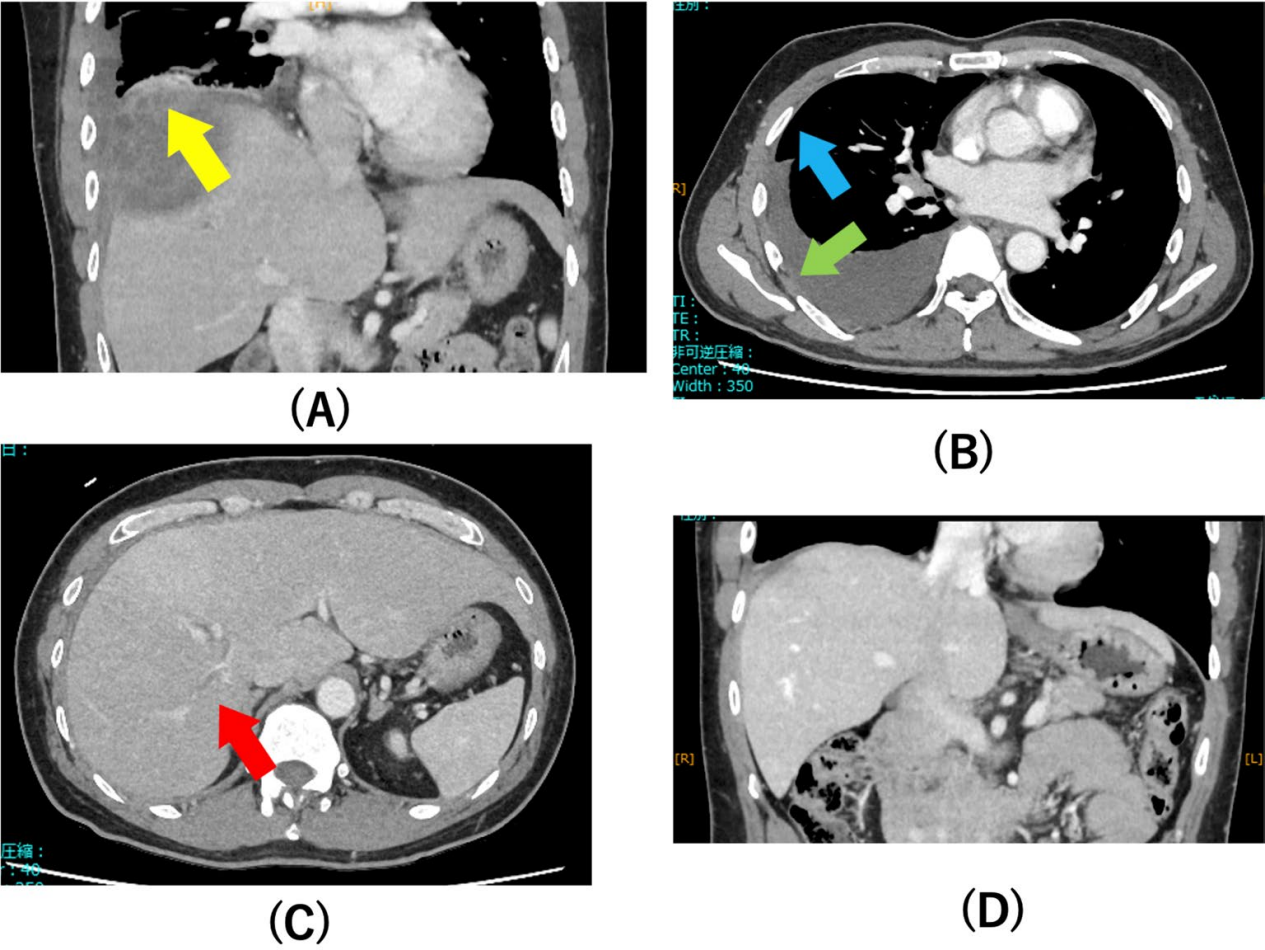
Contrast-enhanced computed tomography (CT) of the thorax and abdomen shows a solitary liver abscess in the right lobe of the liver with loss of diaphragmatic continuity adjacent to the abscess (a, yellow arrow), pleural thickening (b, blue arrow), enlargement of intercostal muscles (b, green arrow) and portal vein branch thrombosis (c, red arrow). CT after resolution shows complete restoration of diaphragmatic rupture (d)
